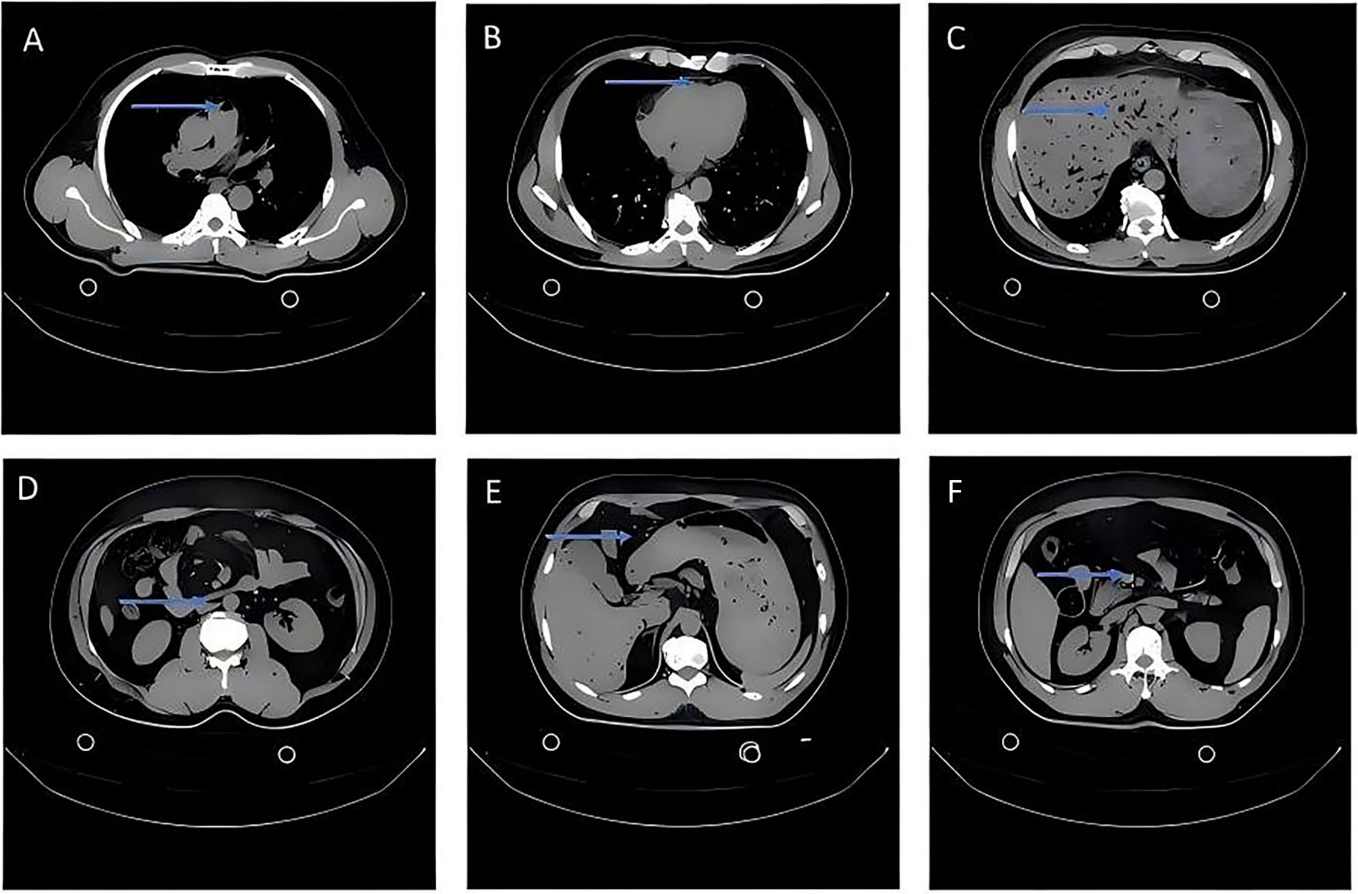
Axial CT images demonstrating air emboli. (A) Gas bubble in the pulmonary artery trunk (arrow). (B) Bubble in the right atrium (arrow). (C) Extensive gas within the intrahepatic portal venous system (arrows). (D) Gas bubble in the inferior vena cava (arrow). (E) Intraperitoneal free gas (arrow). (F) Gas bubble in the superior mesenteric vein (arrow). CT images of chest and abdominal gas emboli (non-contrast, mediastinal window). (A) Gas bubbles in the pulmonary artery trunk (arrow, associated with dyspnea). (B) Gas bubbles in the right atrium (arrow). (C) Extensive gas in the hepatic portal venous system (arrow, potential cause of liver function impairment). (D) Gas bubbles in the inferior vena cava (arrow). (E) Pneumoperitoneum (arrow, may cause abdominal pain). (F) Gas bubbles in the superior mesenteric vein (arrow).
